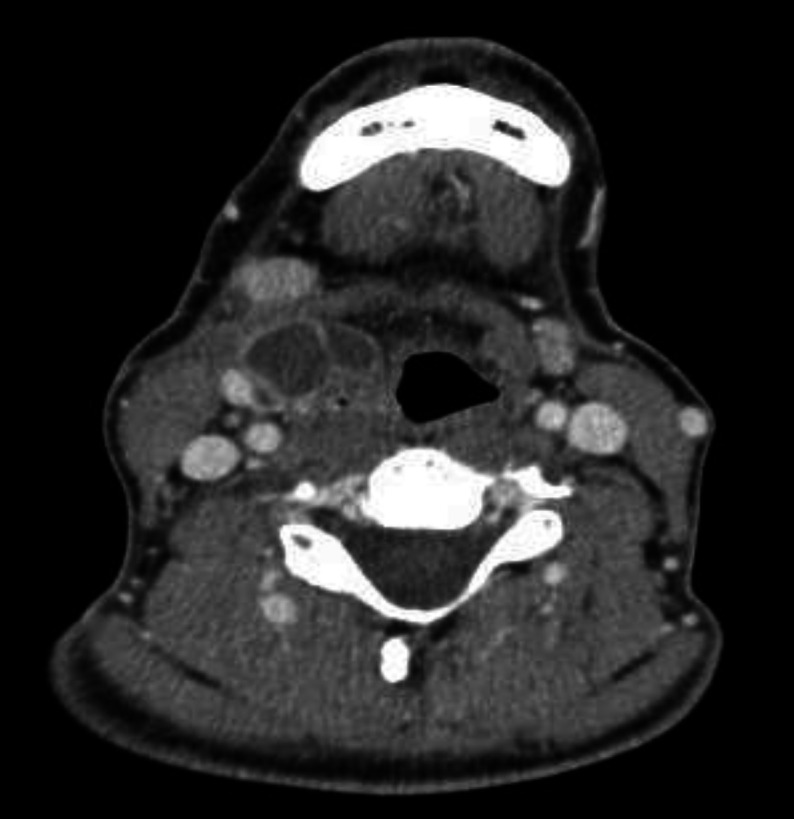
Neck CT scan shows a polylobed collection in right para-laryngeal situs and its anatomical relationships
